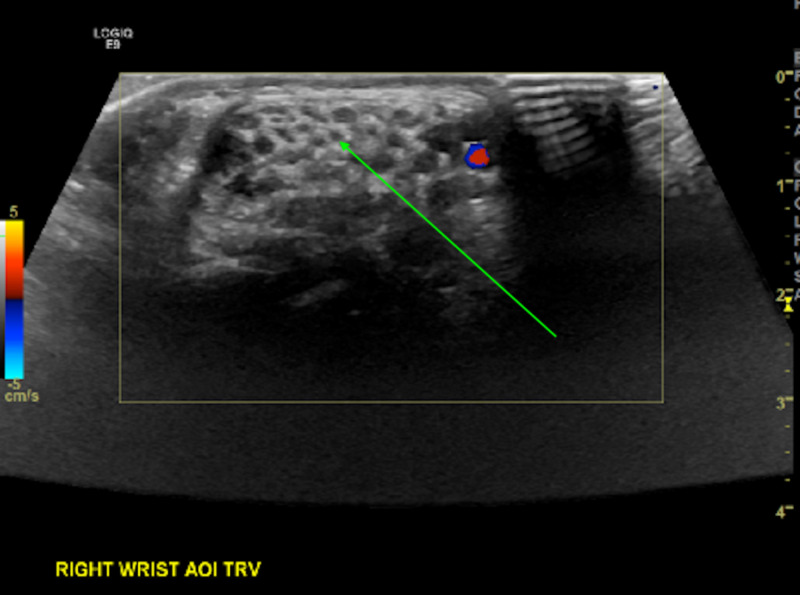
Ultrasound appearance of patient's right wrist showing echogenic fatty tissue interspersed with hypoechoic 'coaxial cabling' identified by the green arrow
